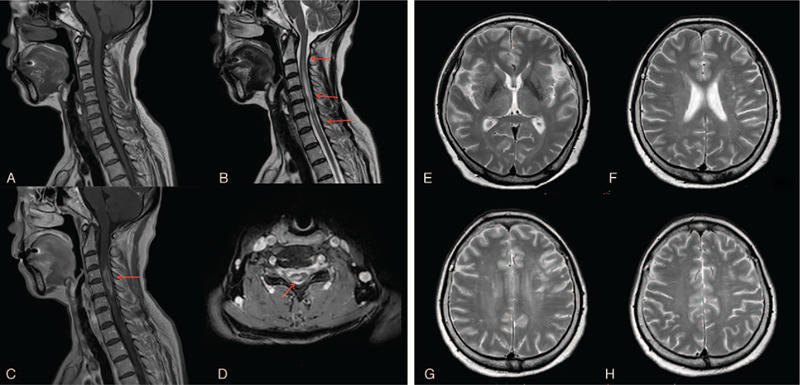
Sagittal T1-weighted (A) T2-weighted (B) images of the spine revealed a longitudinally extensive lesion extending from C2 to C7 vertebrae (red arrows, A–B); an enhanced irregular sign can be observed at the C3 to C5 level (red arrow, D). Axial cervical magnetic resonance imaging (MRI) images reveal a mass lesion mainly located in the center of the cord (red arrow, C). Initial brain MRI results confirmed the absence of any abnormality prior to intravenous methylprednisolone treatment (E–H).
